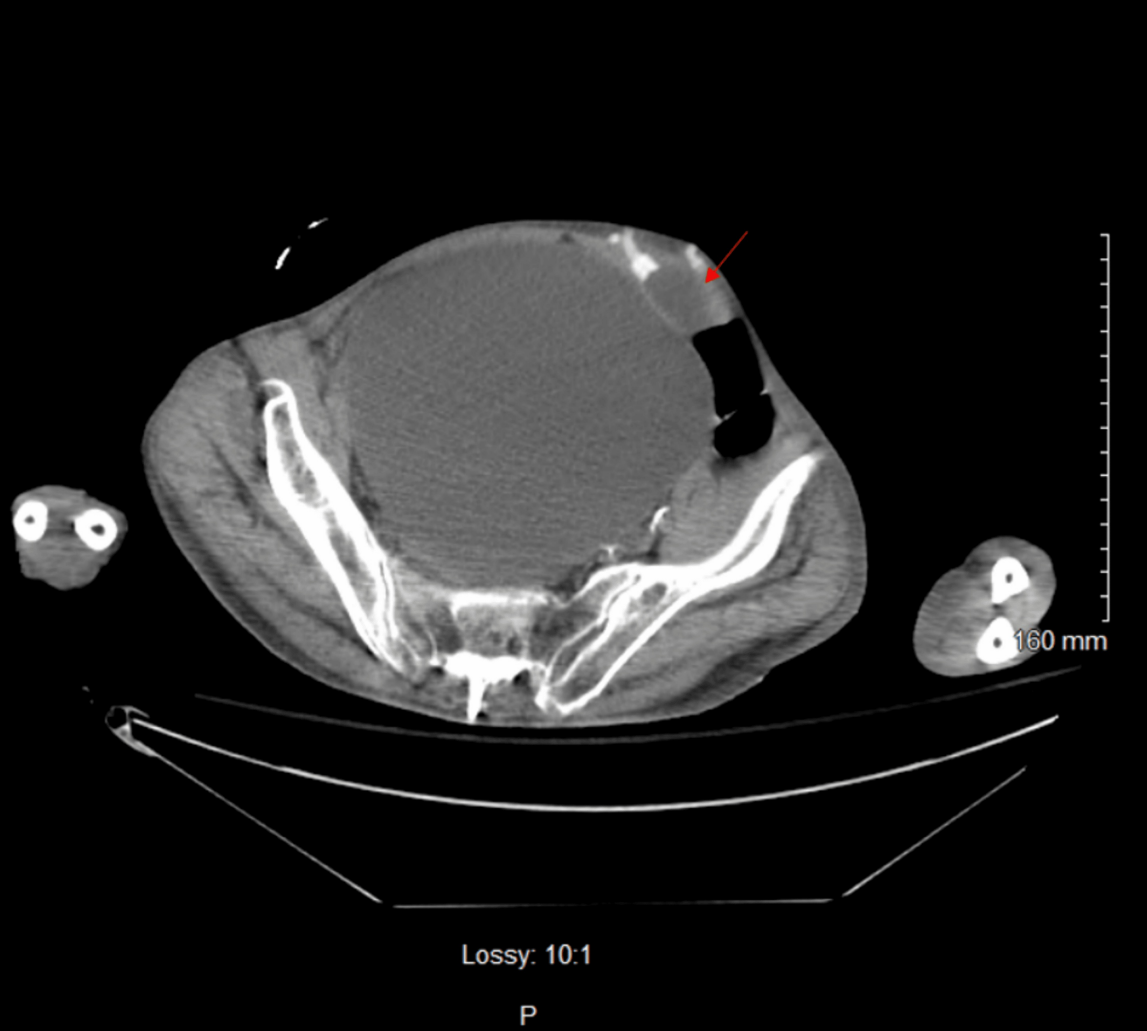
Axial non-contrast CT image at the bladder level The bladder is obstructed and distended, measuring 18 cm, with no apparent gross thickening of the bladder wall; a red arrow points to the abdominal balloon reservoir of the artificial urethral sphincter.
